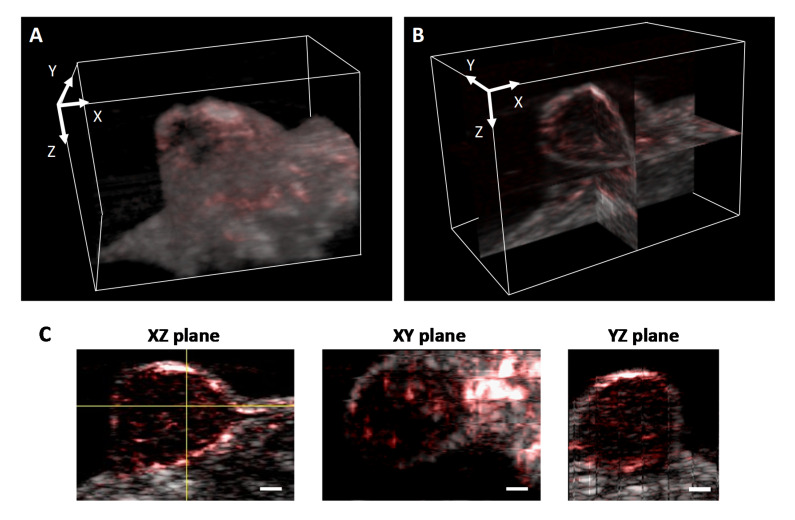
(A) Overlay of ultrasound and photoacoustic images of tumors reconstructed in 3D (Video 2) and (B) in orthoslice view. (C) Tumor images displayed in different 2D planes. (Scale bar = 2 mm, Video S2, MOV, 779 kB).
